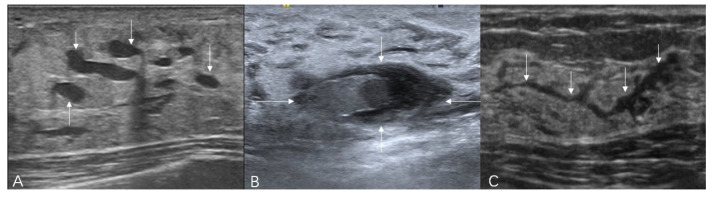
Ultrasound features of non-mass breast lesions of Type II. (A) Ultrasound shows dilated breast ducts, which may be a normal finding in late pregnancy and lactation (indicated by the white arrows); (B) a case of intraductal papilloma. Ultrasound reveals that the solid components within the dilated duct have a relatively regular shape (indicated by the white arrows); (C) a case of DCIS. Ultrasound shows irregular dilation of the breast ducts (indicated by the white arrows).
